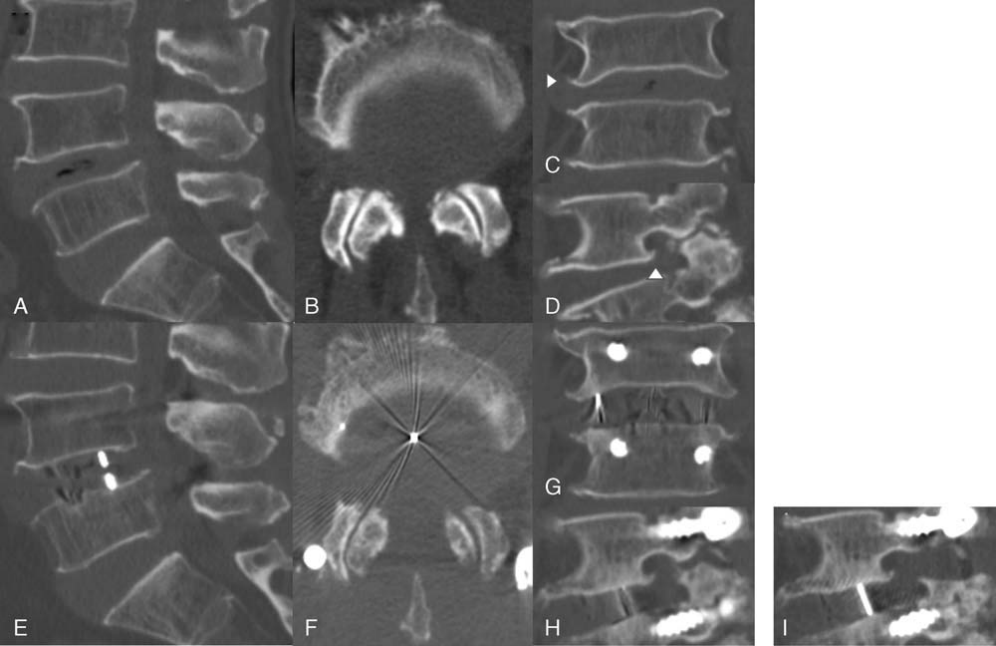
Lumbar CT images before oblique lumbar interbody fusion in February 2018 (A–D) and 10 weeks after the first surgery in April 2018 (E–H) and after the second surgery (I). We measured and compared the anterior, middle, and posterior disc heights in the sagittal plane. We also compared the foraminal height by measuring the vertical distance from the top of the foramen to the superior end plate of the L5 body. The white arrowheads point to the osteophytes we focused on. A: sagittal plane, B: axial plane, C: coronal plane, D: right foraminal view. E: sagittal plane, F: axial plane, G: coronal plane, H: right foraminal view. The height of the anterior, middle, and posterior discs increased by 10% (16.17– 17.73 mm), 32% (13.87–18.26 mm), and 6% (10.95–11.56 mm), respectively, while the height of the right foramen increased by13% (19.36–21.93 mm). Figure 2I presents the right foraminal view after right facetectomy and right foraminotomy, it can be confirmed that the lamina and calcification tissues around the foramen were clearly removed.
